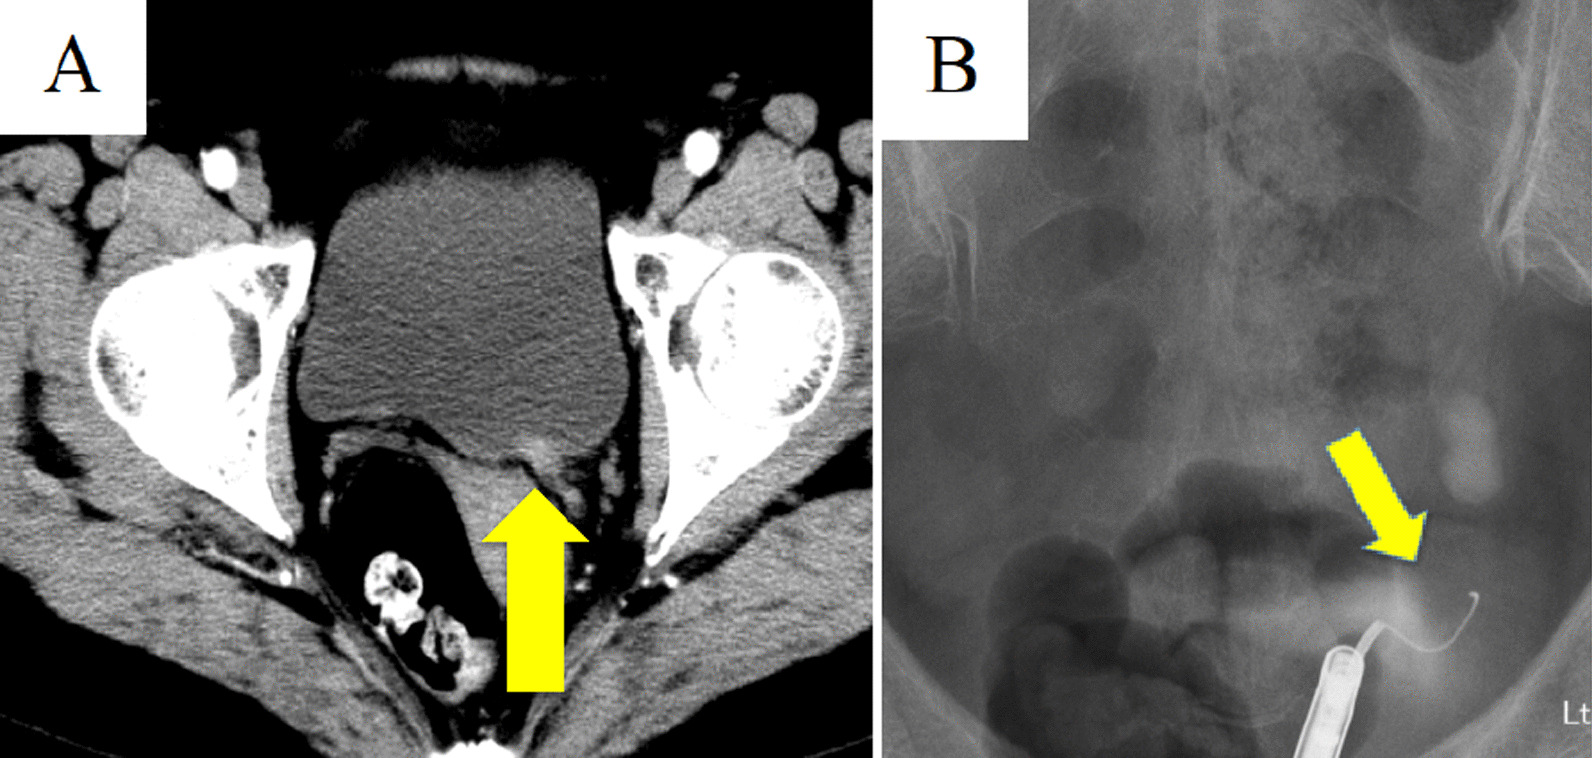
a Enhanced computed tomography showed enhanced thick wall in the lower ureter (tumor: arrow). b Left lower ureter was completely stenosed in retrograde pyelography (tumor: arrow)
